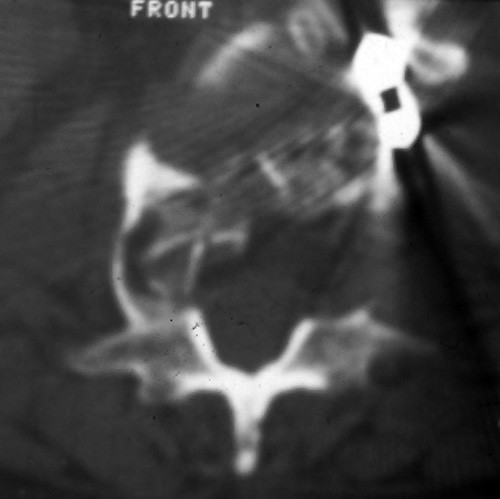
Postoperative CT scan revealed no residual spinal stenosis at the L2 level.
